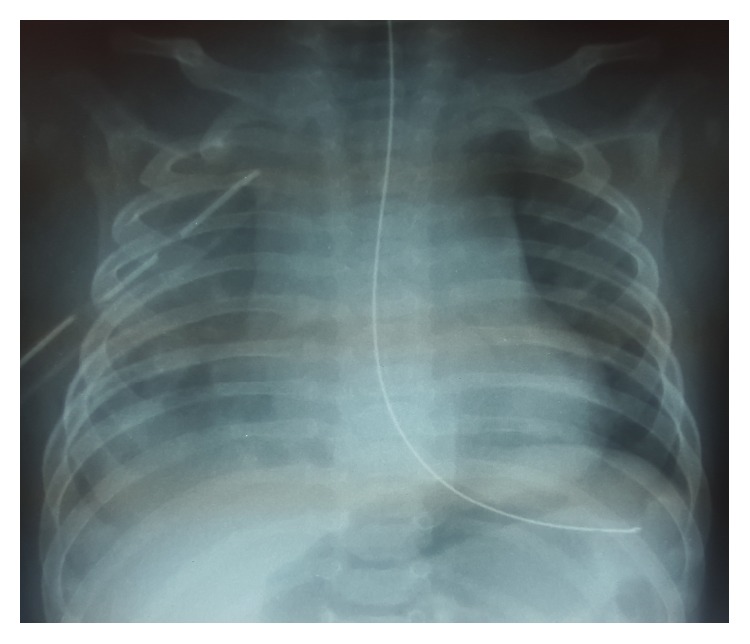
Chest X-ray after chest drainage.
